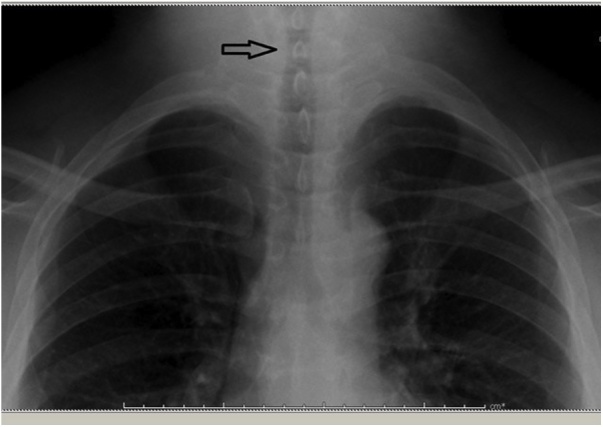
Chest x-ray shows the ovalization of the tracheal lumen (arrow).
